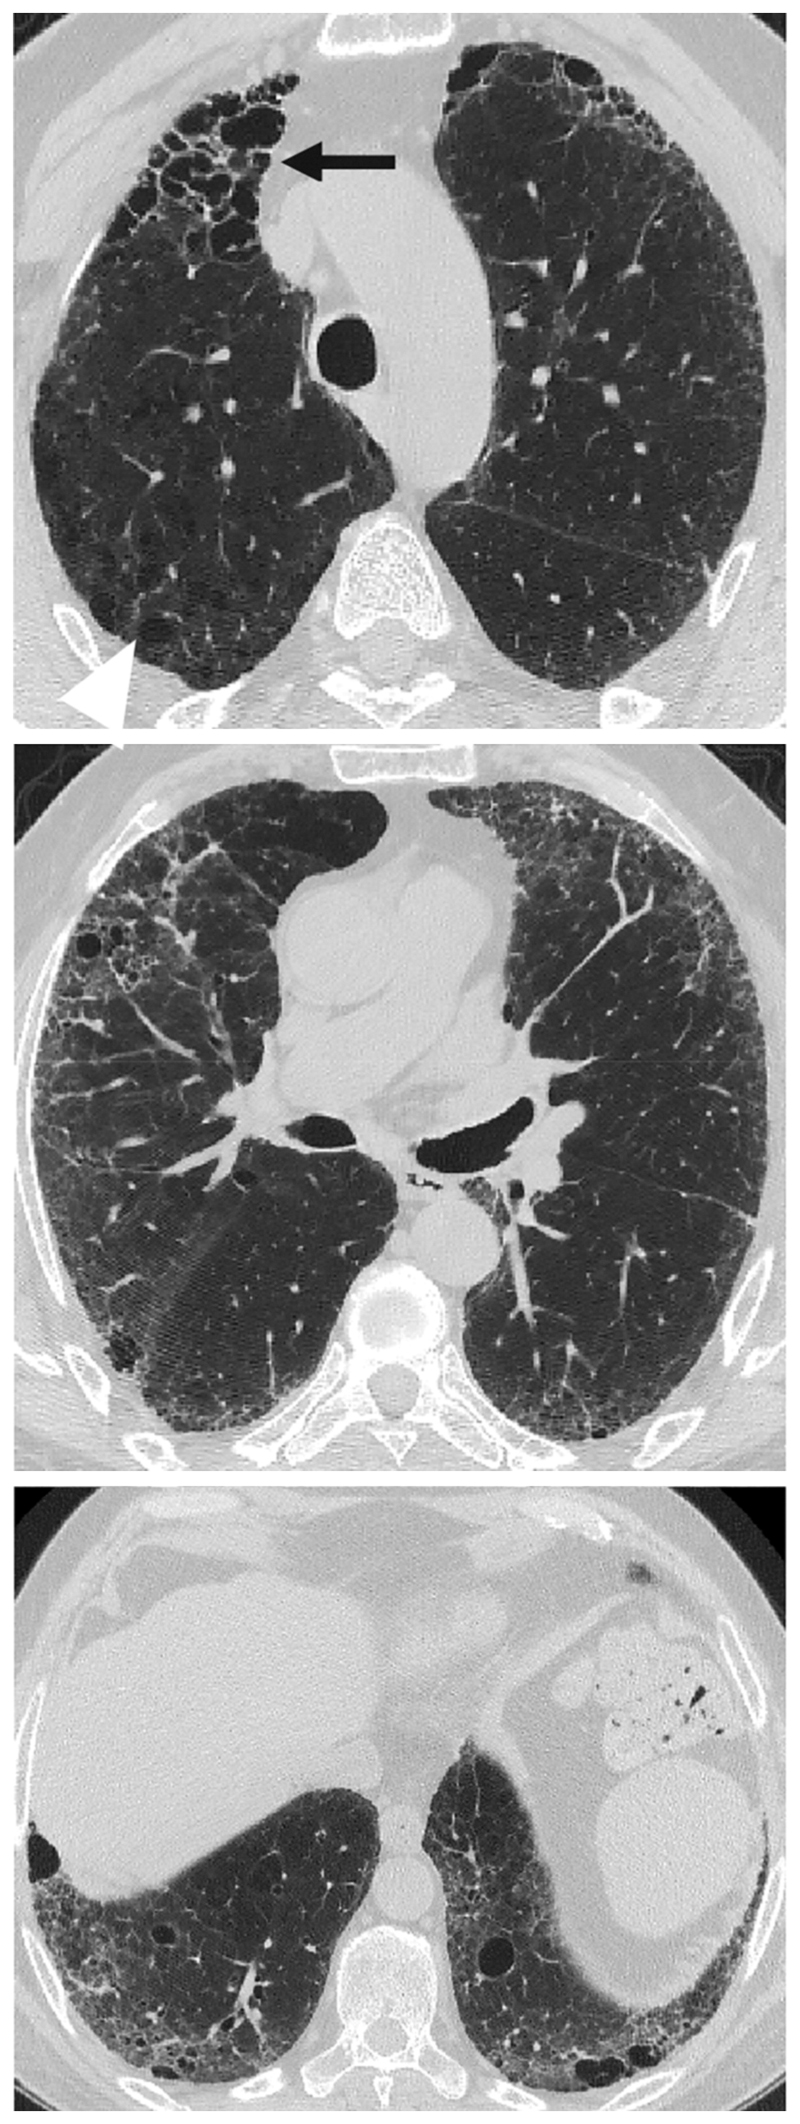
HRCT in a 69-year-old male with idiopathic pulmonary fibrosis, showing diffuse emphysema through the lung zones and lower zone predominant fibrosis (combined pulmonary fibrosis and emphysema, unclassifiable pattern). Emphysema in the right upper lobe is a combination of admixed (black arrow) and isolated emphysema (white arrowhead). Admixed emphysema is visible in the midzones, whilst in the lower zones a mixture of admixed and isolated paraseptal and centrilobular emphysema is apparent.
